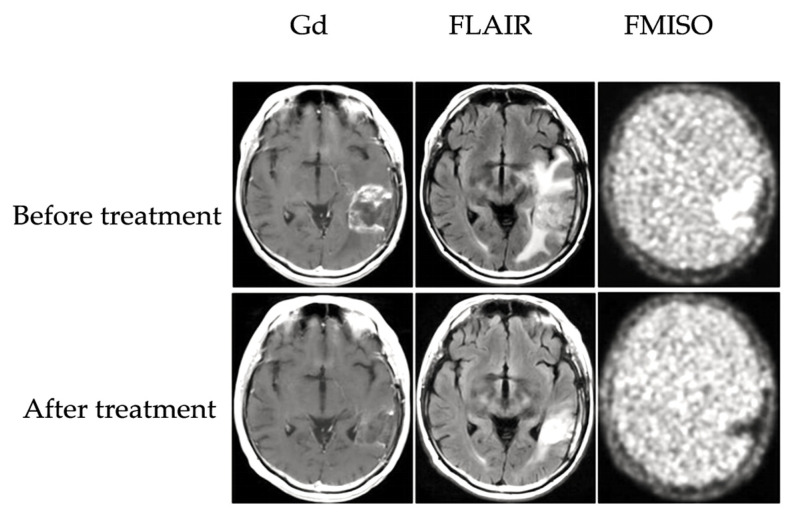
Representative MRI and FMISO-PET images of a recurrent glioma patient before and after bevacizumab treatment. Gadolinium-enhanced MRI and FLAIR MRI show morphological changes, while FMISO-PET highlights hypoxic regions with decreased tracer uptake after treatment. Adapted from Hanley R et al. [55].
